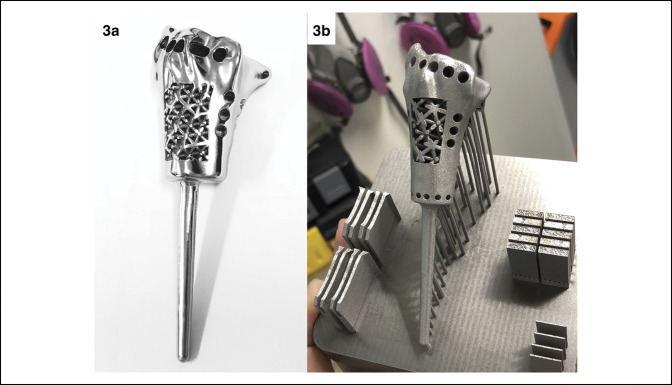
Figures demonstrating a custom-made distal radial endoprosthesis. A, Designed preoperatively by our developed technique via three-dimensional CT. B, Postprocessing model.
